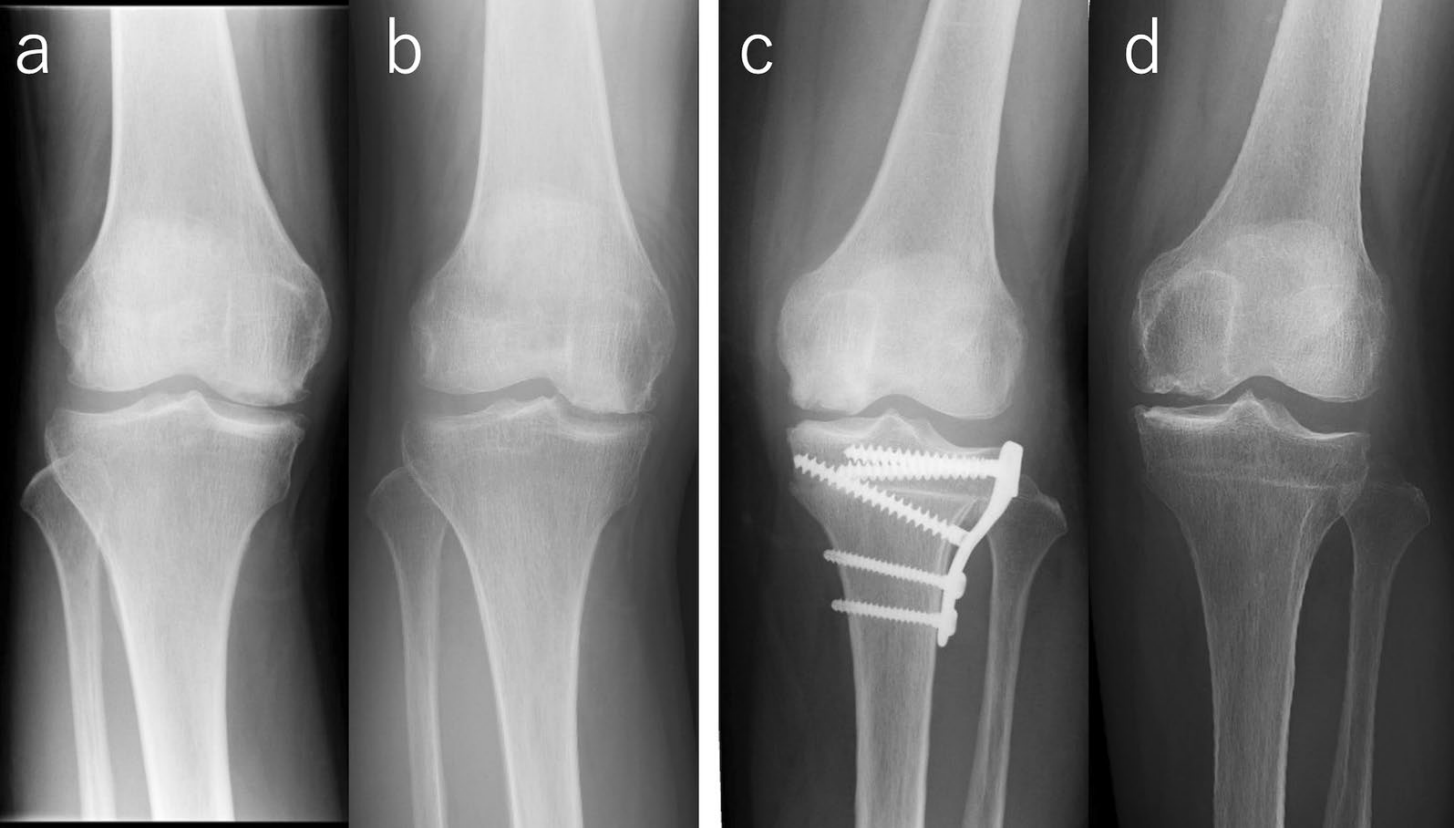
Postoperative (a, c) and final follow-up (b, d) radiographs of (a, b) a 53-year-old female patient with OAT whose FTA changed from 179.0° to 180.5° at 14.2 years after surgery, and who subsequently underwent revision TKA; and (c, d) a 63-year-old female patient with OAT + HTO whose FTA remained unchanged at 168.5° at 19.3 years after surgery
